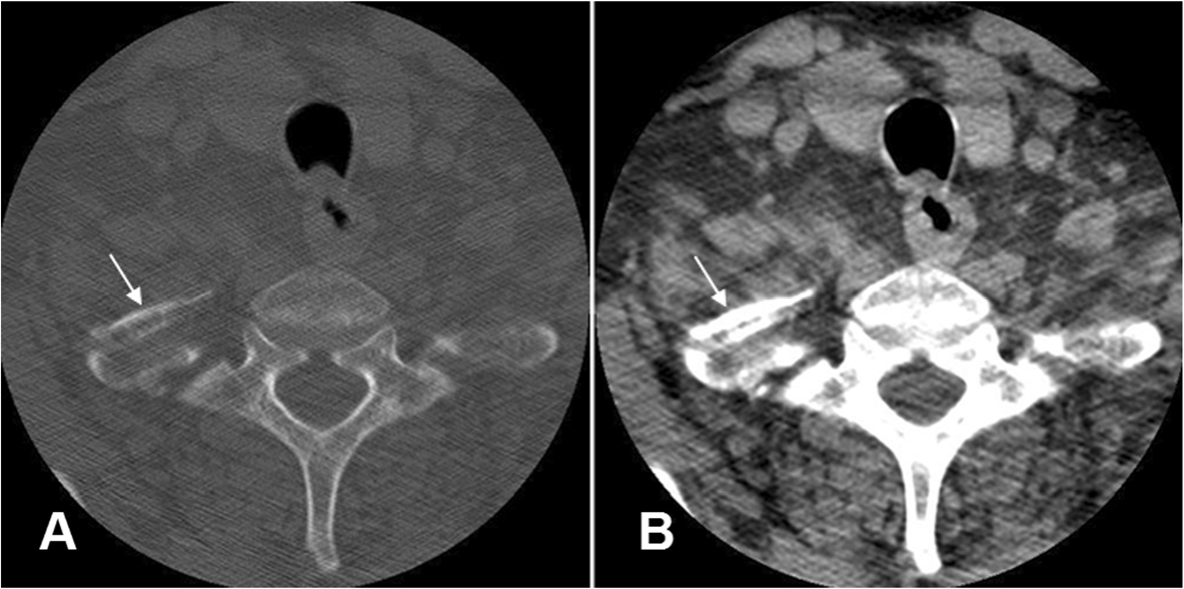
CT of the thoracic spine with contrast. The CT bone window image (A) and CT soft tissue window (B) reveal an anteriorly displaced rib fracture of the proximal right first rib (white arrows).
